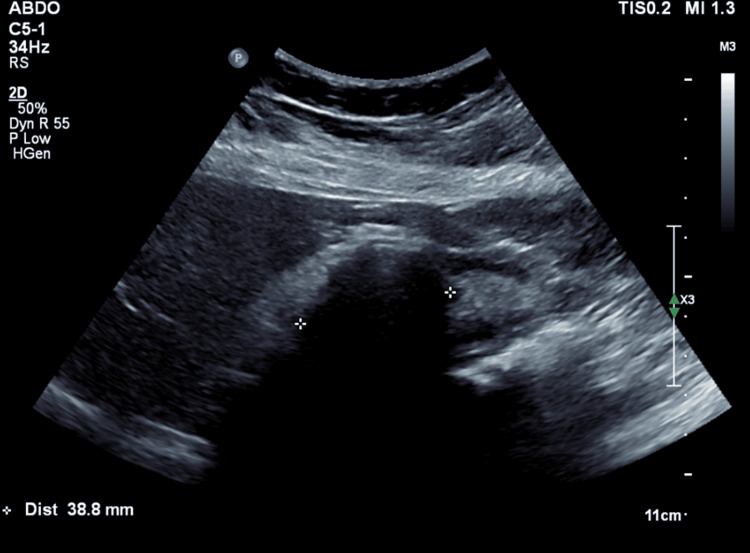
Ultrasound demonstrating a large 39 mm gallstone in the gallbladder, there is an acoustic shadowing typical of gallstones.
